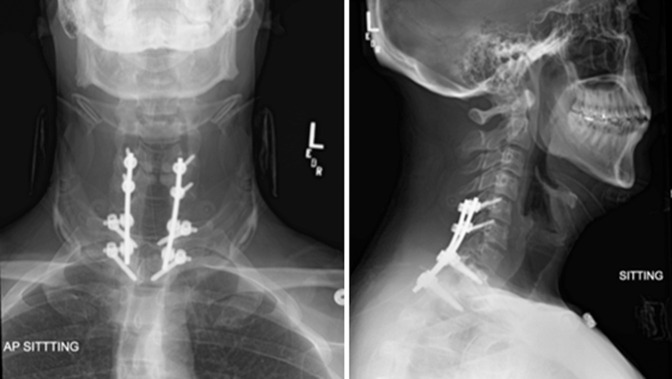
Postoperative AP and lateral cervical spine radiographs demonstrating posterior spinal instrumentation from C5 to T2.
